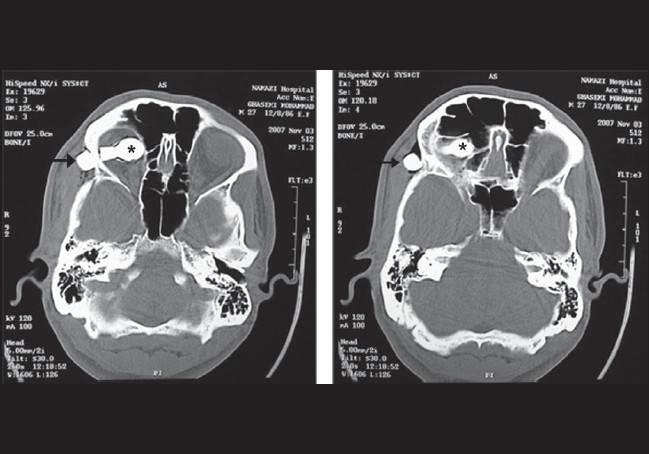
Orbital computed tomography shows two parts of foreign body: The short part (asterisk) located in the superior orbit, and the tip of the long part (arrow)
